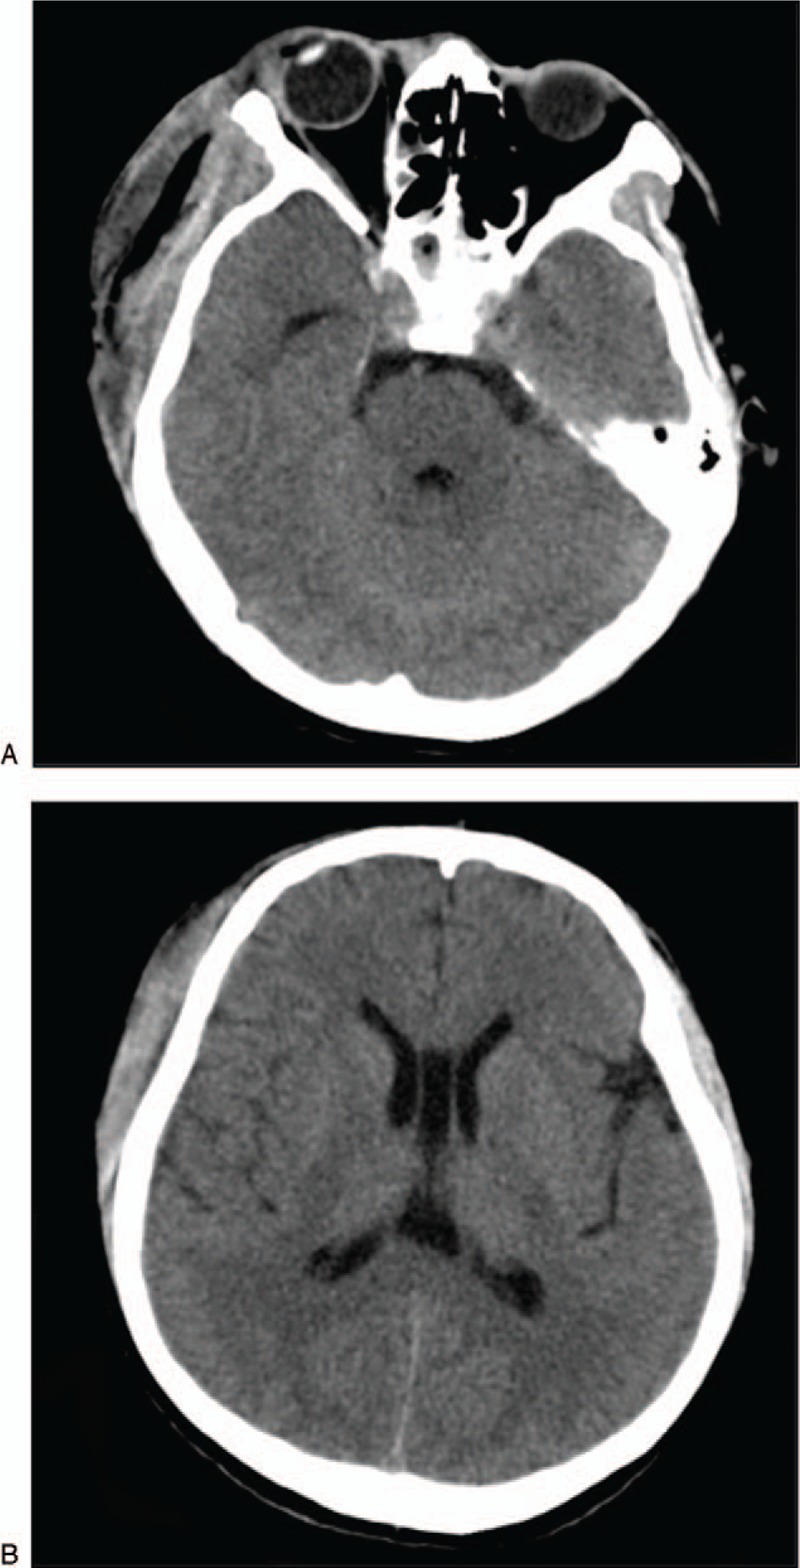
(A and B) Computed tomography shows the ventricle size returns to normal.
